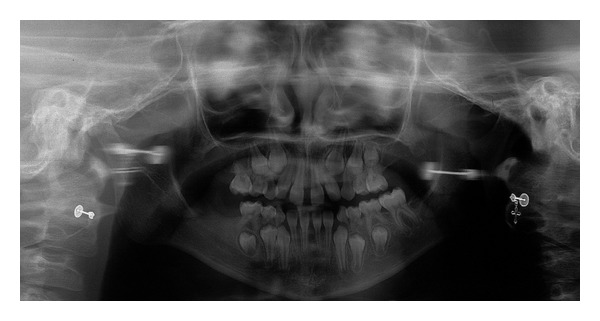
Panoramic radiograph demonstrating absence of teeth.
